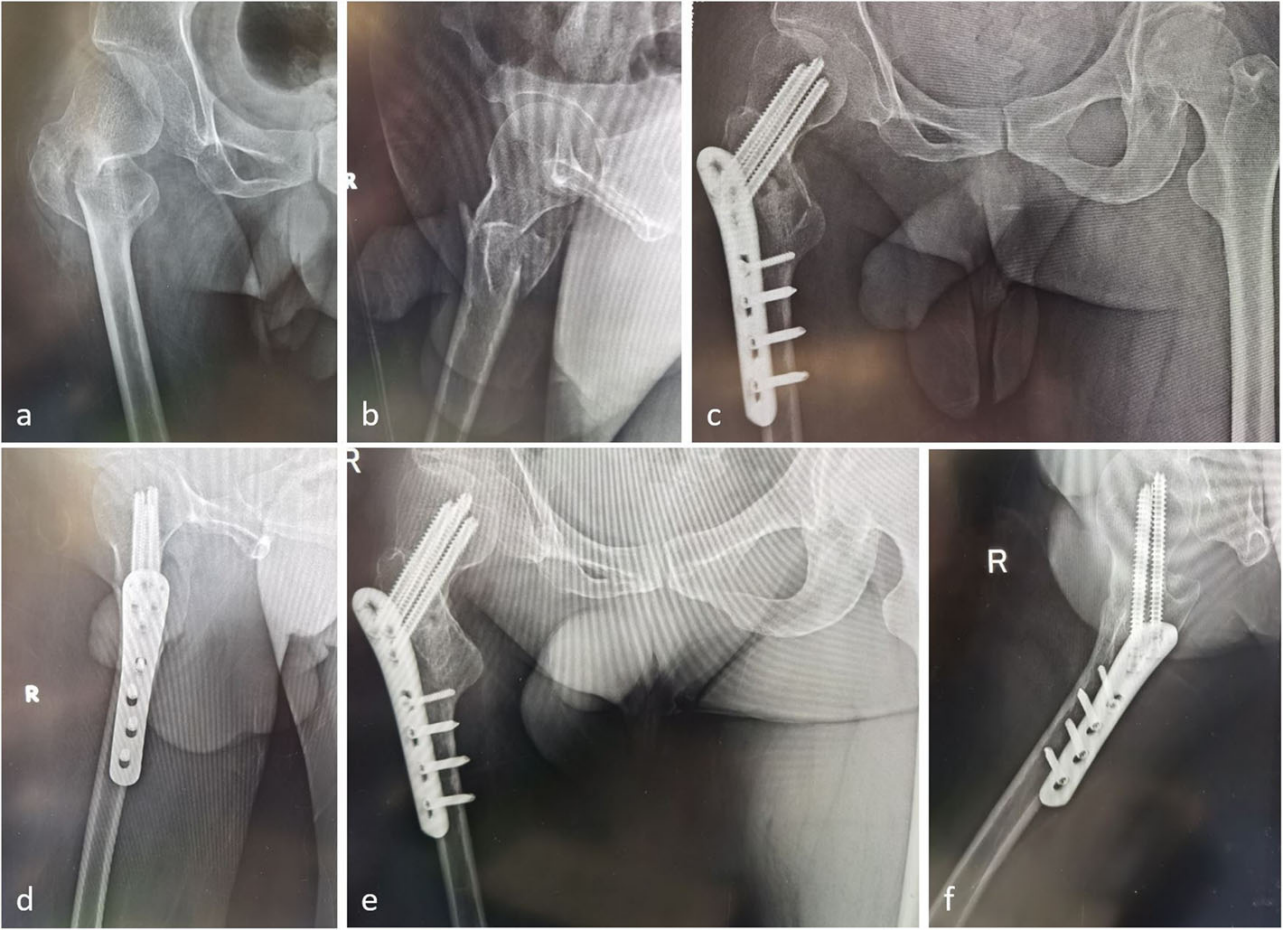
The patient was a 56-year-old male who accidently fell from a standing height causing right hip pain and limited activity, while past medical history indicated poliomyelitis sequela that had been present for decades. A preoperative anteroposterior X-ray (a) and a lateral X-ray (b) showed a right femoral intertrochanteric fracture. Postoperative X-ray radiographs of the anteroposterior (c) and lateral view (d) 7 days after surgery. X-ray radiographs of the anteroposterior (e) and lateral (f) 17 months after surgery indicated fracture union
